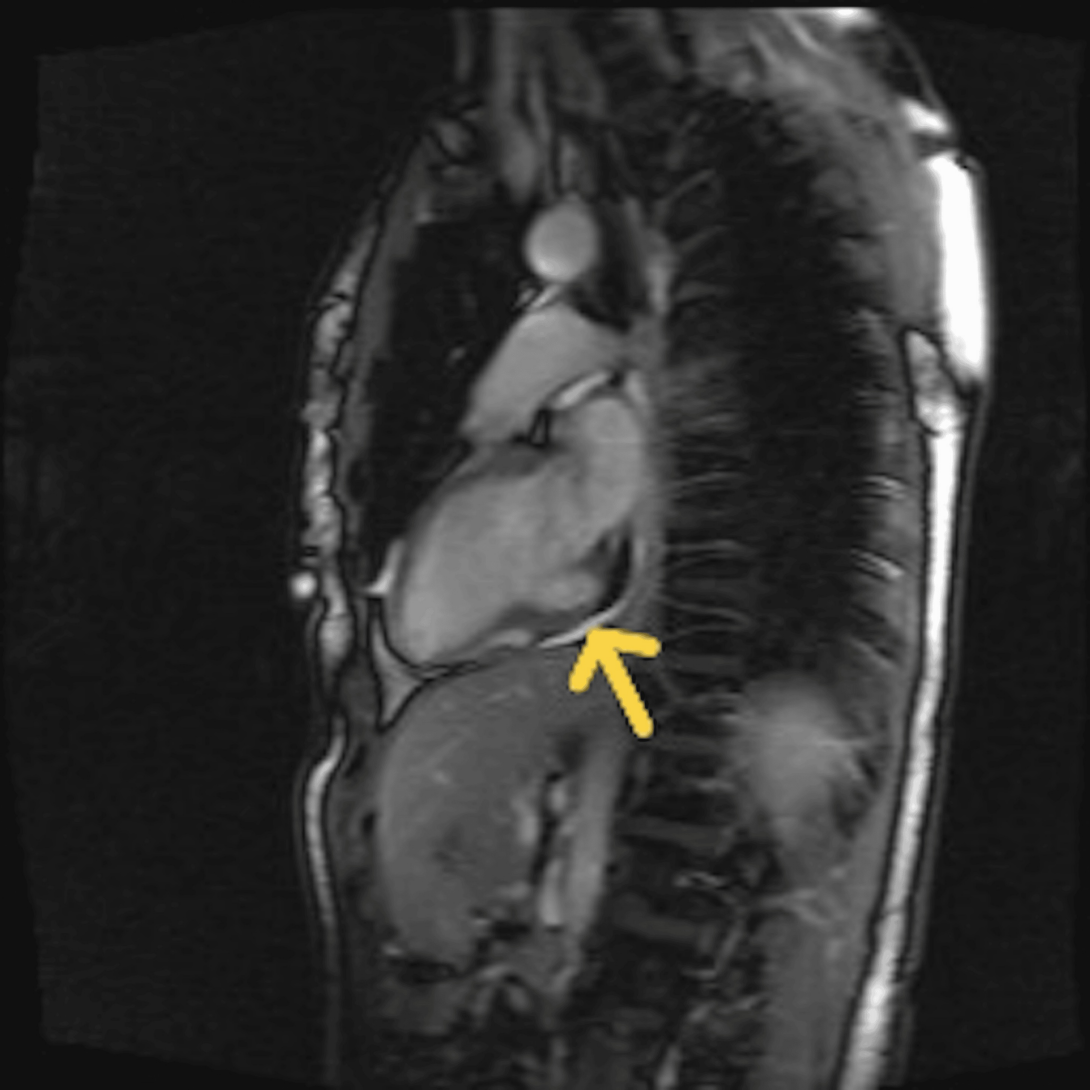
Cardiac magnetic resonance imaging (cMRI) showing cardiac diverticulum in the base of left-ventricular inferior wall during diastole (yellow arrow).
